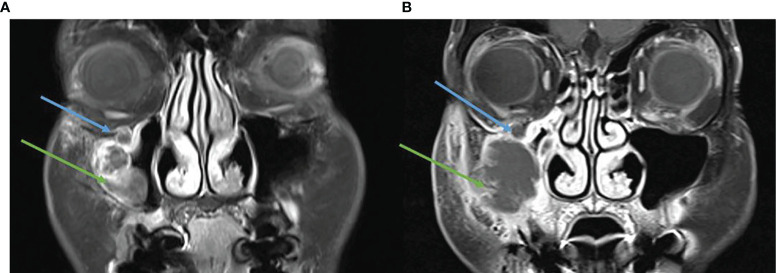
(A) Baseline, left, MRI post contrast T1 Fat Sat demonstrating a destructive lesion involving the anterior wall of the right maxillary sinus (green arrow) and perineural spread in the right infraorbital nerve (blue arrow). (B) MRI post contrast T1 Fat Sat, post three cycles of cemiplimab 250 mg/3-weekly, enlarging destructive mass and persisting perineural spread.
